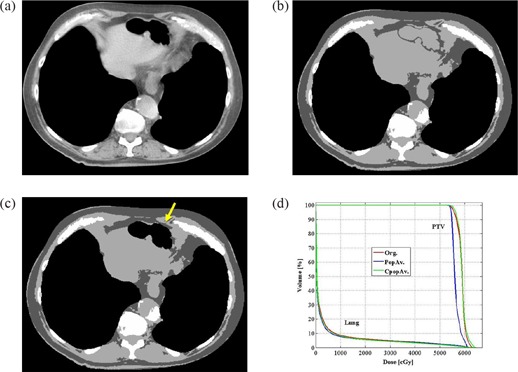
An example of a patient with a hiatus hernia filled with a large amount of gas. original treatment planning CT image (mediastinum window); (b) treatment planning CT image of the same slice as 5a with the 5 bulk densities applied; (c) treatment planning CT image of the same slice as 5a. and b. where the air density is applied to hiatus hernia (yellow arrow); (d) DVH comparing the PTV and lung of the 3 plans.
